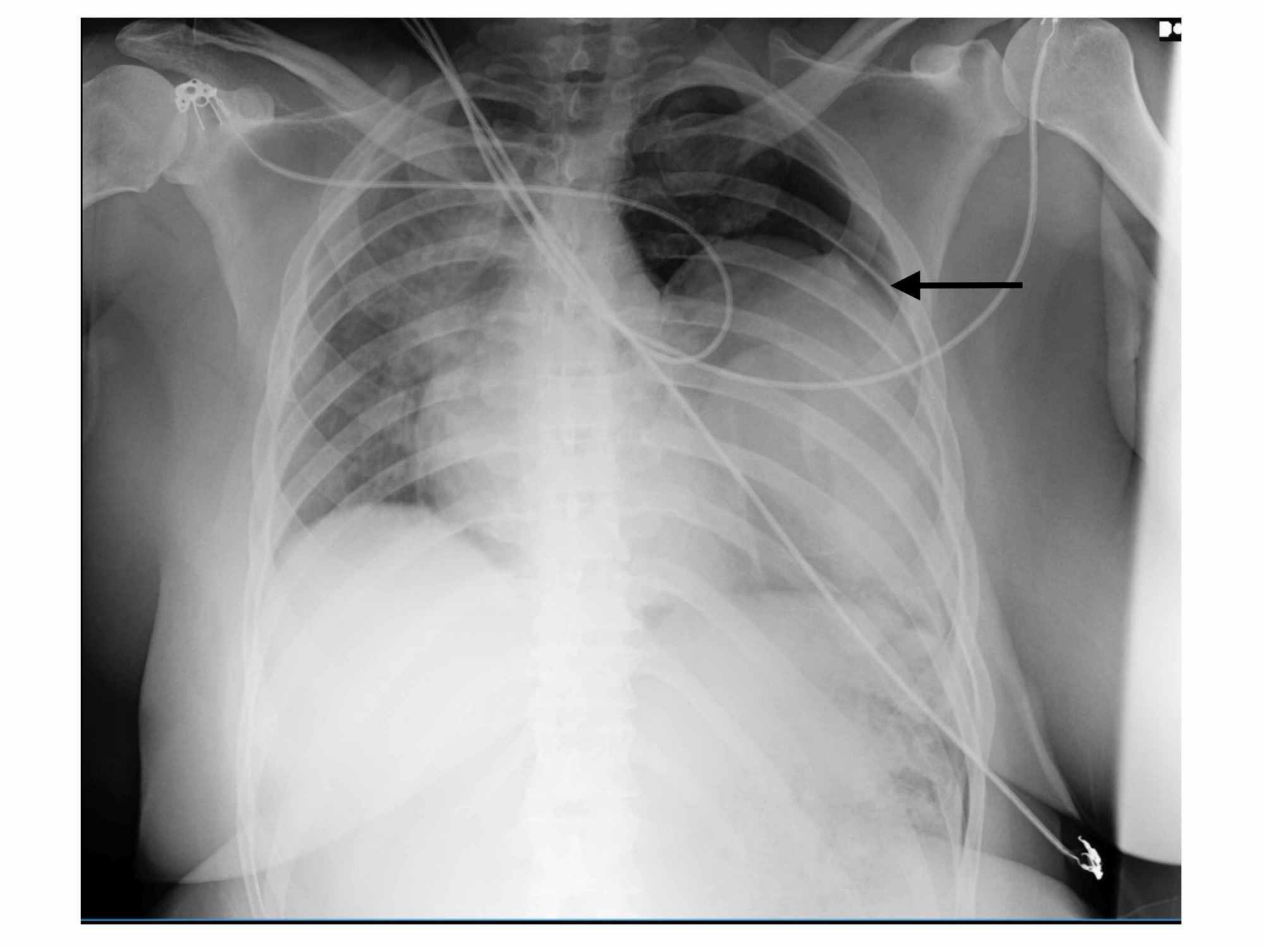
X-ray showing a left pneumothorax and a suspicious gastric shadow in left hemi-thorax
